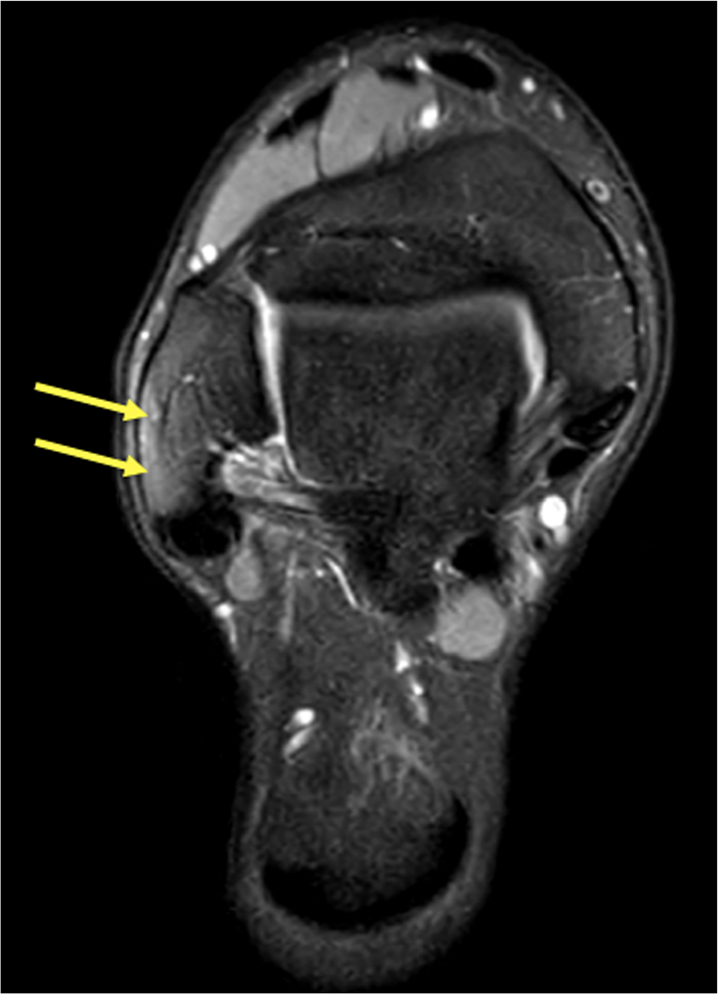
An 18-year-old runner with Achilles tendon pain. MRI showed a typical artifact in the lateral malleolus on an FS sequence (arrows). There were no changes in the Achilles tendon
